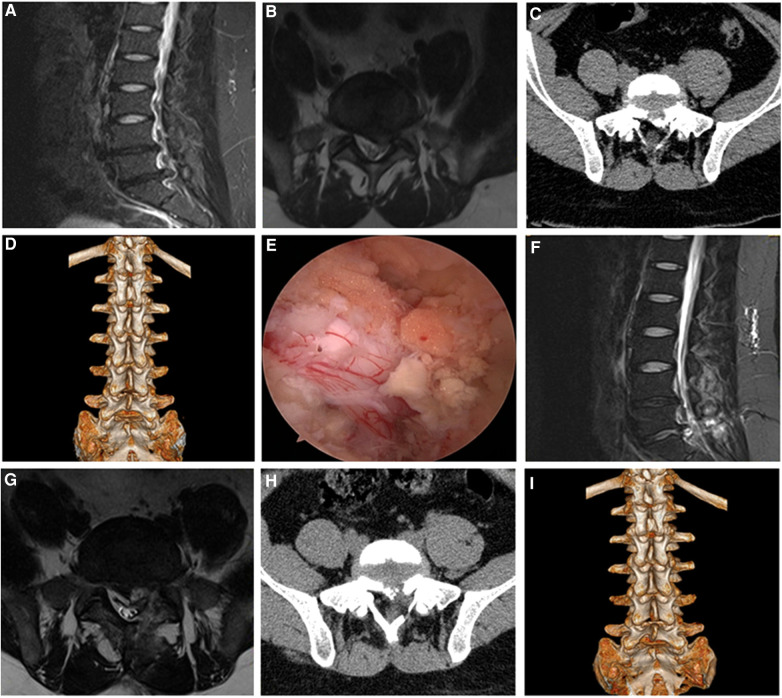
Male, 28 years old, L5/S1 lumbar disc herniation with posterior apophyseal ring separation. (A) Preoperative sagittal MR image showed L5/S1 lumbar disc herniation; (B) Preoperative axial MR image showed herniated lumbar disc compressed nerve root and dural sac; (C) Preoperative axial CT image showed separated bony fragment of vertebral body; (D) Preoperative 3D-CT image; (E) Intraoperative image after complete neural decompression; (F) Postoperative sagittal MR image revealed the complete decompression of the spinal canal; (G) Postoperative axial MR image showed the complete removal of herniated disc and bony fragment; (H) Postoperative axial CT image showed the removal of the bony fragment; (I) Postoperative 3D-CT image showed the lamina window and preservation of the facet joints.
